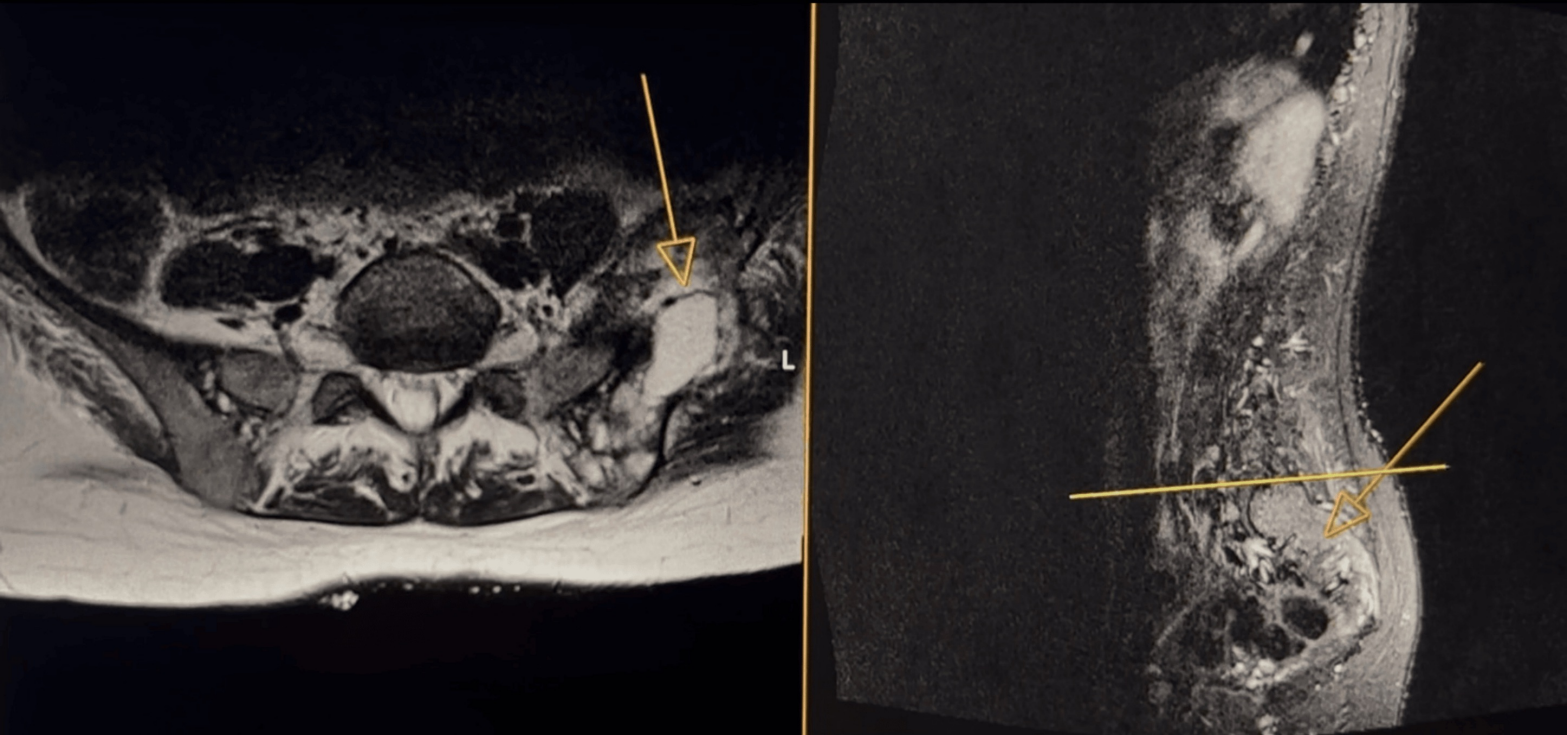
Magnetic resonance imaging of the spine and pelvis confirming soft tissue infiltration and a lytic lesion in the left pelvis
